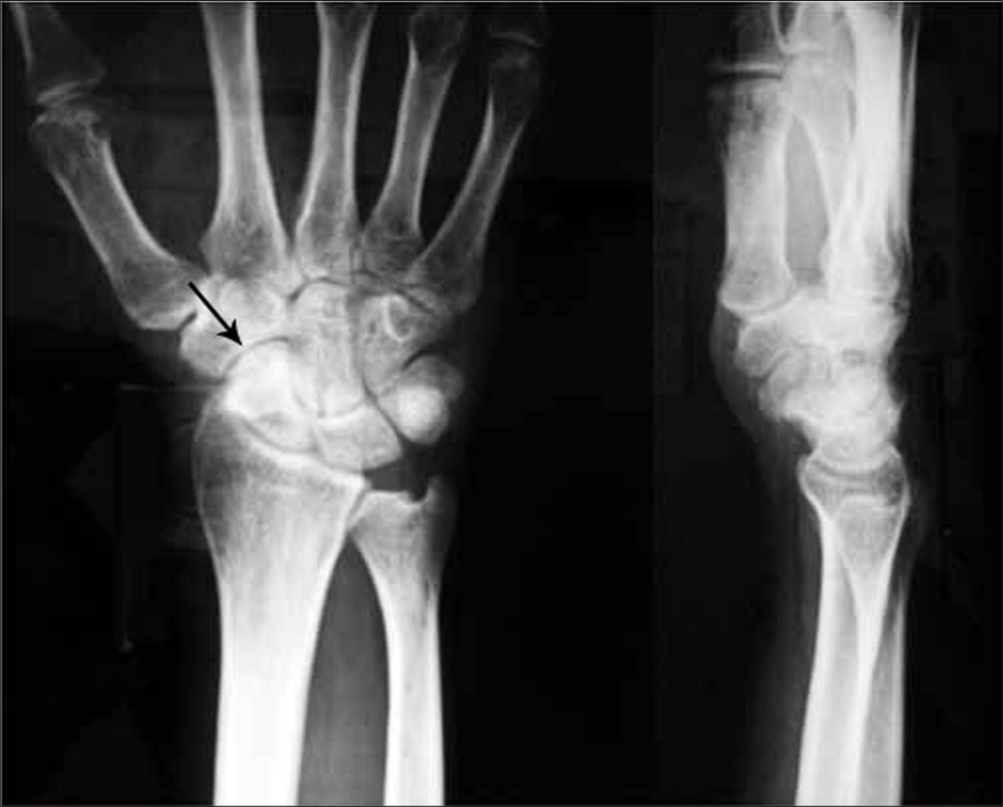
Anteroposterior and lateral radiograph of wrist showing increased density of distal pole of scaphoid (arrow) suggestive of avascular necrosis
